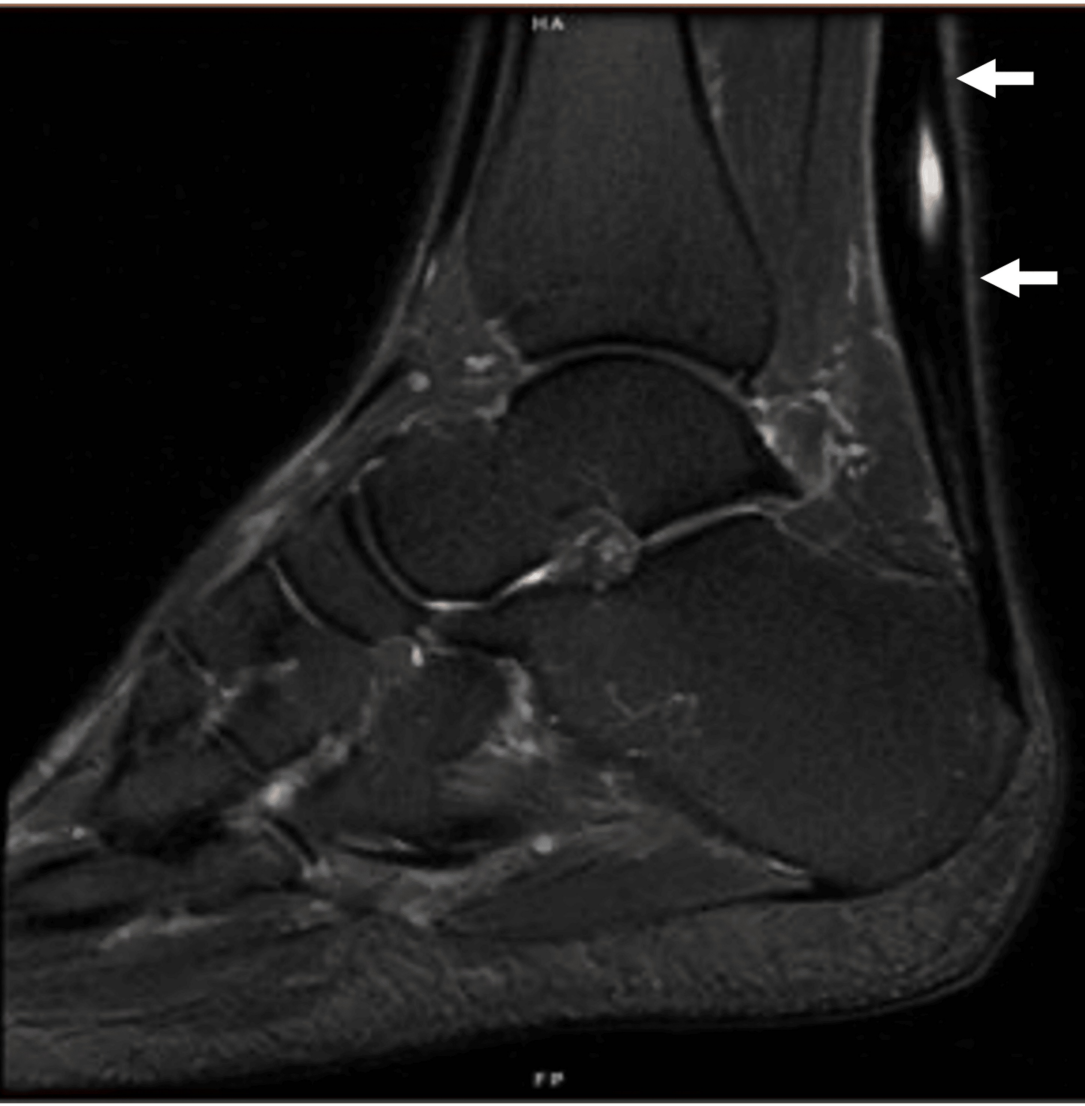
Sagittal fat-suppressed T2 MRI of the ankle showing mild Achilles tendinosis with an interstitial tear. Arrows: Achilles tendon.
